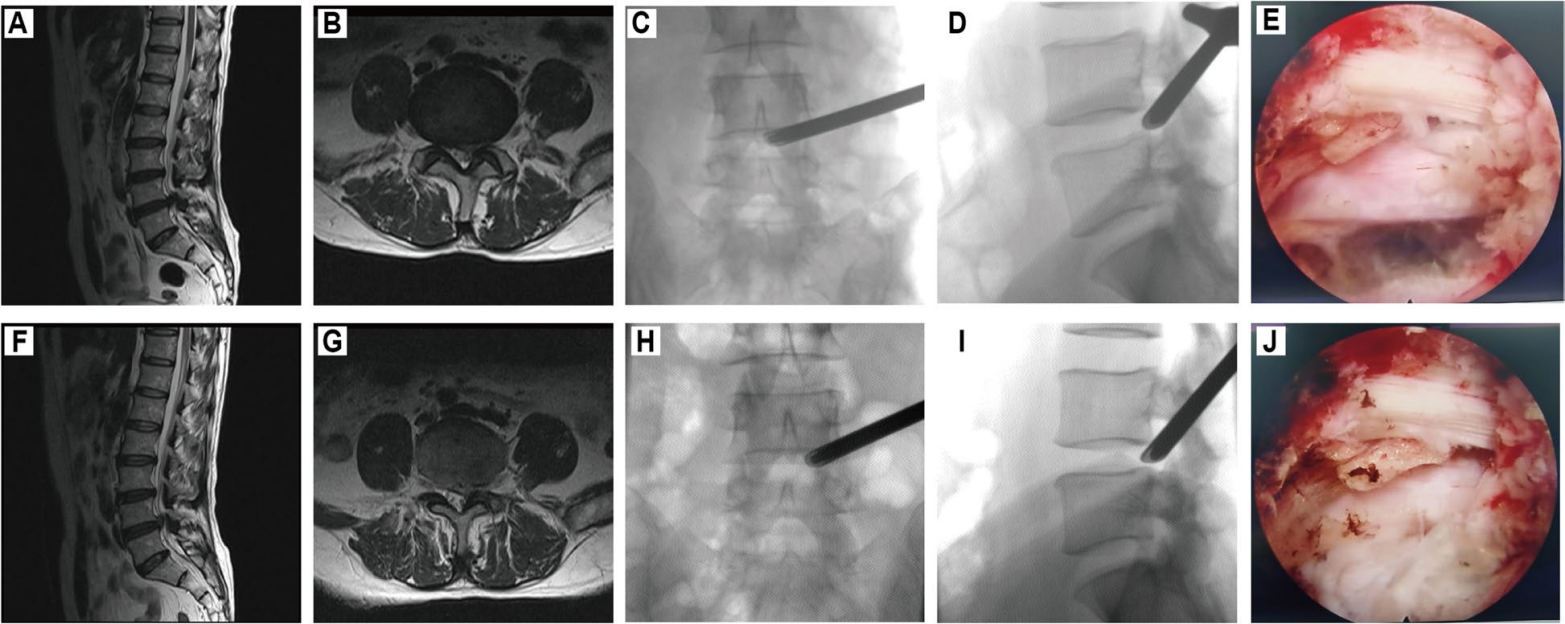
TESSYS for recurrent lumbar disc herniation. A, B Preoperative MRI of the initial surgery: L4/5 disc herniated. C, D The fluoroscopic trajectory of working cannula for removing intracanalicular herniated disc in the initial surgery. E The traversing nerve root is free after removal of the intracanalicular herniated disc in the initial surgery. F, G Preoperative MRI of the second surgery: L4/5 disc herniated. H, I The fluoroscopic trajectory of working cannula for removing intracanalicular herniated disc in the second surgery. J The traversing nerve root is free after removal of the intracanalicular herniated disc in the second surgery
